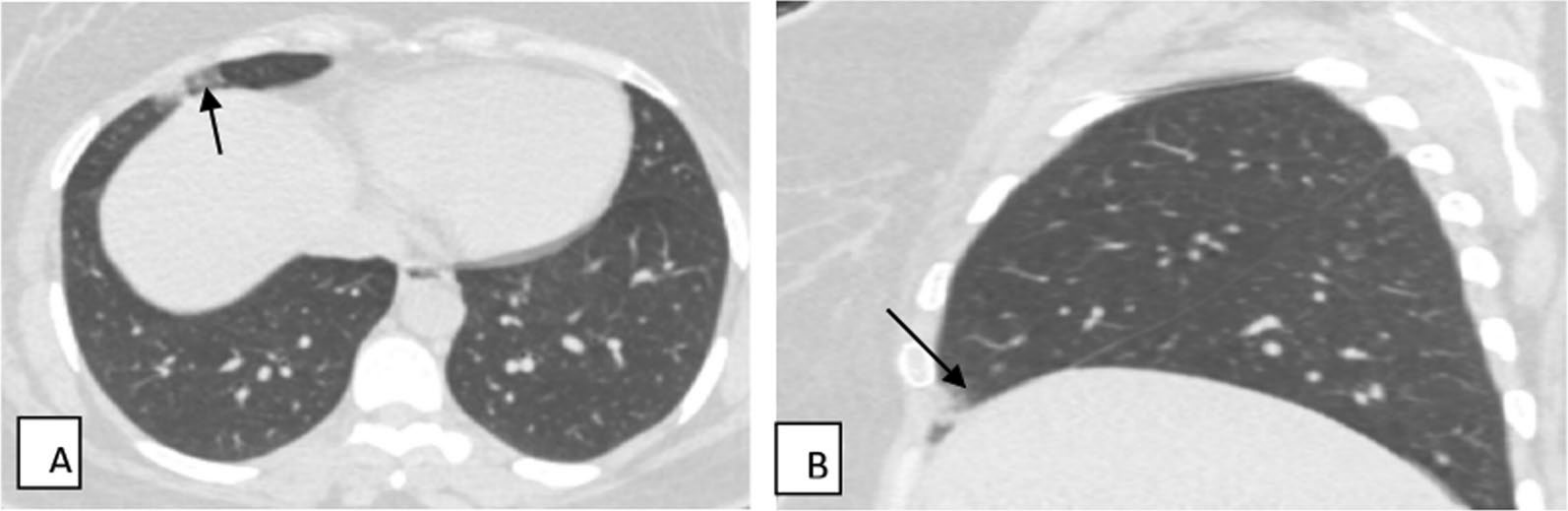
Female patient aged 34 years old presented by loss of smell and taste only. Axial (A) and coronal (B) CT cuts show single patchy area of ground-glass density is seen at right middle lobe (black arrow)
